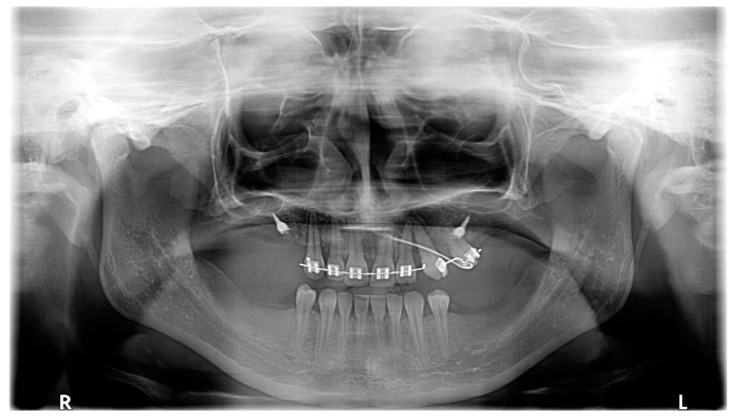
Panoramic radiograph performed after orthodontic extrusion.
